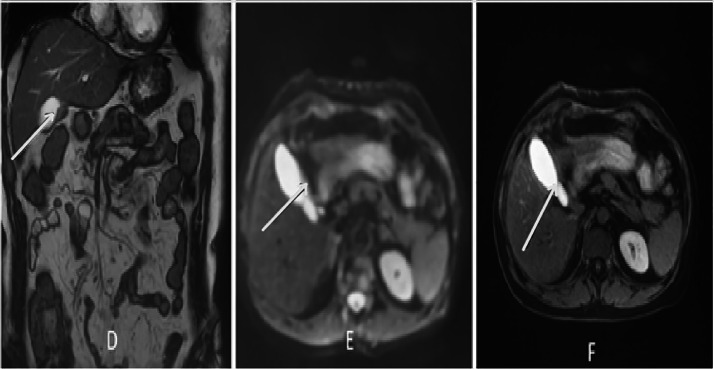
MRI images in coronal T2 section (D), DWI axial section (E) and T1 axial section after gadolinium injection (F), showing a parietal lesion of the gallbladder, protruding endoluminal, in T2 and DWI hyper signal, taking up gadolinium after injection (white arrows).
